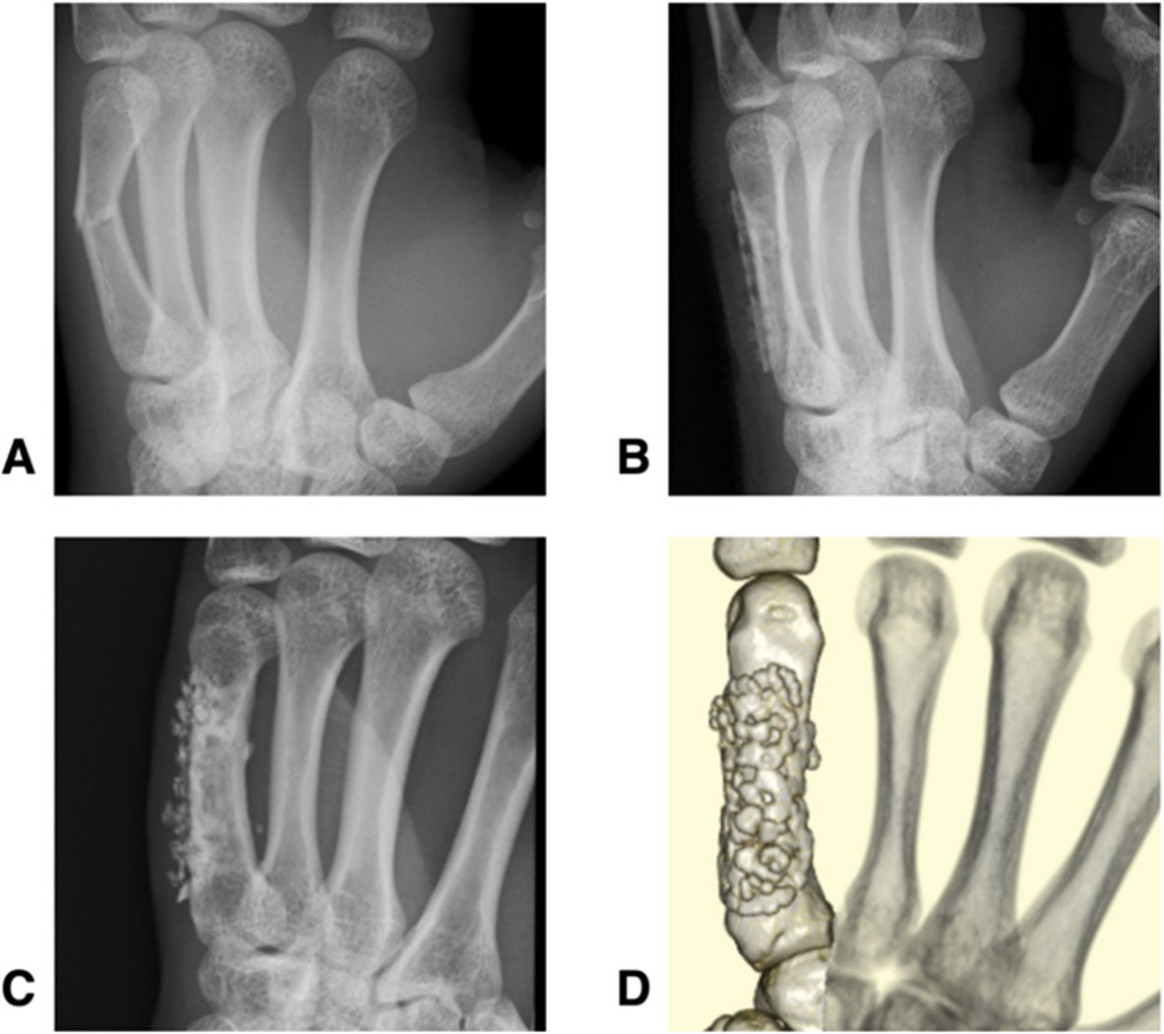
Radiographic images of a repaired transverse metacarpal fracture of the left little finger in a 16-year-old male (Case 1). The preoperative radiograph (a), postoperative radiograph (b), and final follow-up radiograph (c), which was taken 5 years 8 months post-surgery are pictured. A final follow-up 3D-computed tomography image (d) is also shown
